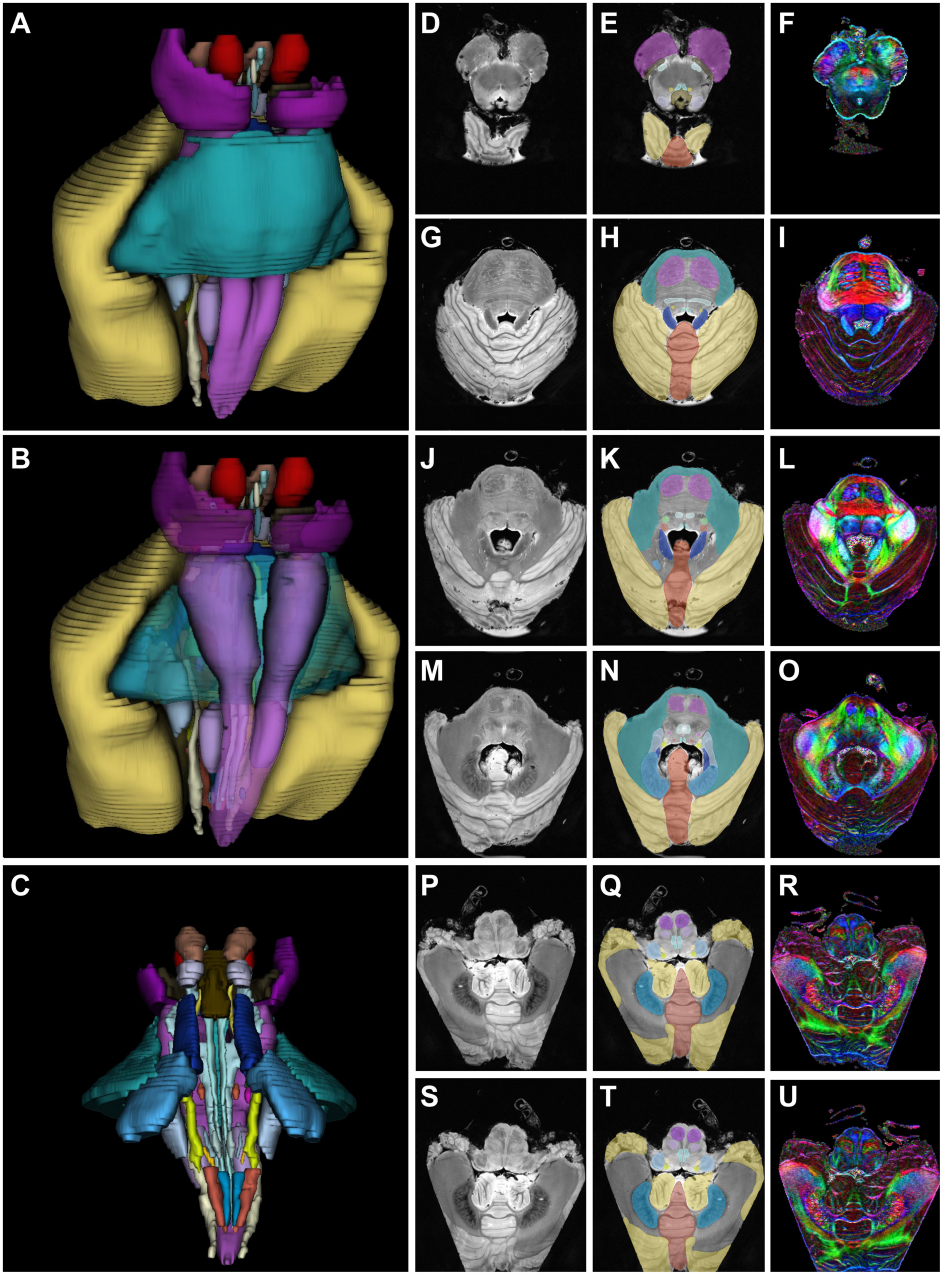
3D reconstruction of segmented structures. Segmentation and color maps of high-resolution fractional anisotropy axial MR images. (A, D–I) Mesencephalon. (B, J–O) Pons. (C, P–U) Medulla. (D, G, J, M, P, S) show MR images, (E, H, K, N, Q, T) show segmented structures, and (F, I, L, O, R, U) show fractional anisotropy images of the mesencephalon through the medulla. *Used with permission from Barrow Neurological Institute, Phoenix, Arizona*.
